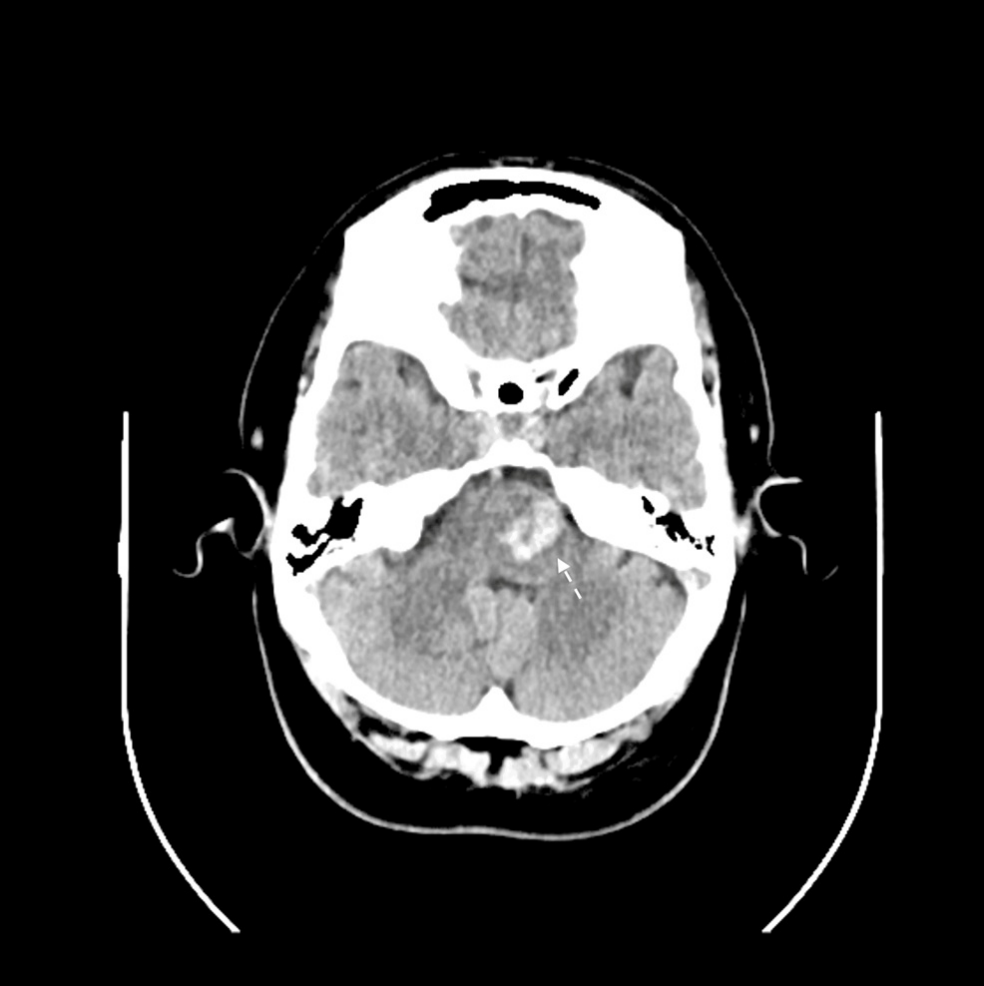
CT scan of the brain showing left pontine hemorrhage.
